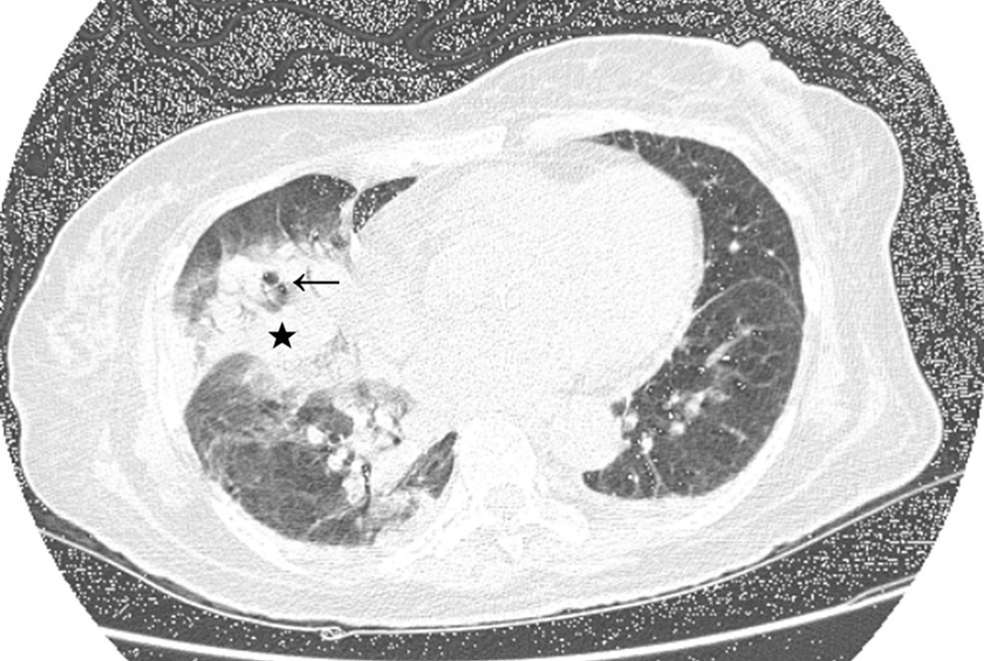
High-resolution CT of the thorax showing an increase in the size of the previous consolidation (black star) and presence of air bronchograms (black arrow).
